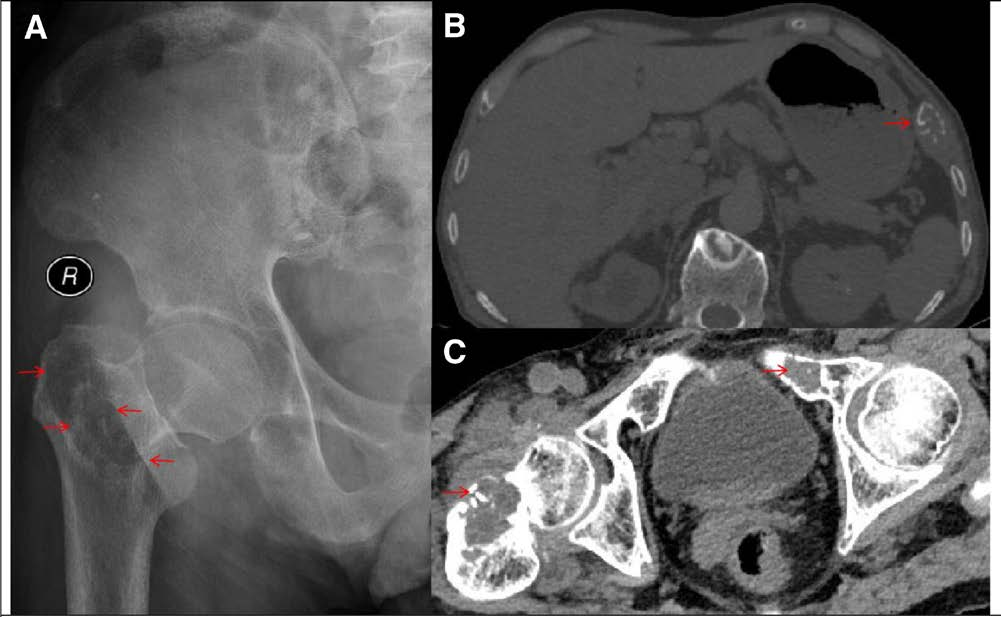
(A) The preoperative anteroposterior showed radiolucent appearance and femoral neck fracture (red arrows); (B) The pulmonary CT scan showed a obvious costal lytic lesion (red arrow); (C) The pelvic CT showed femoral neck fracture and upper branch of pubis with suspicious bone lesions (red arrows). CT = computed tomography.
